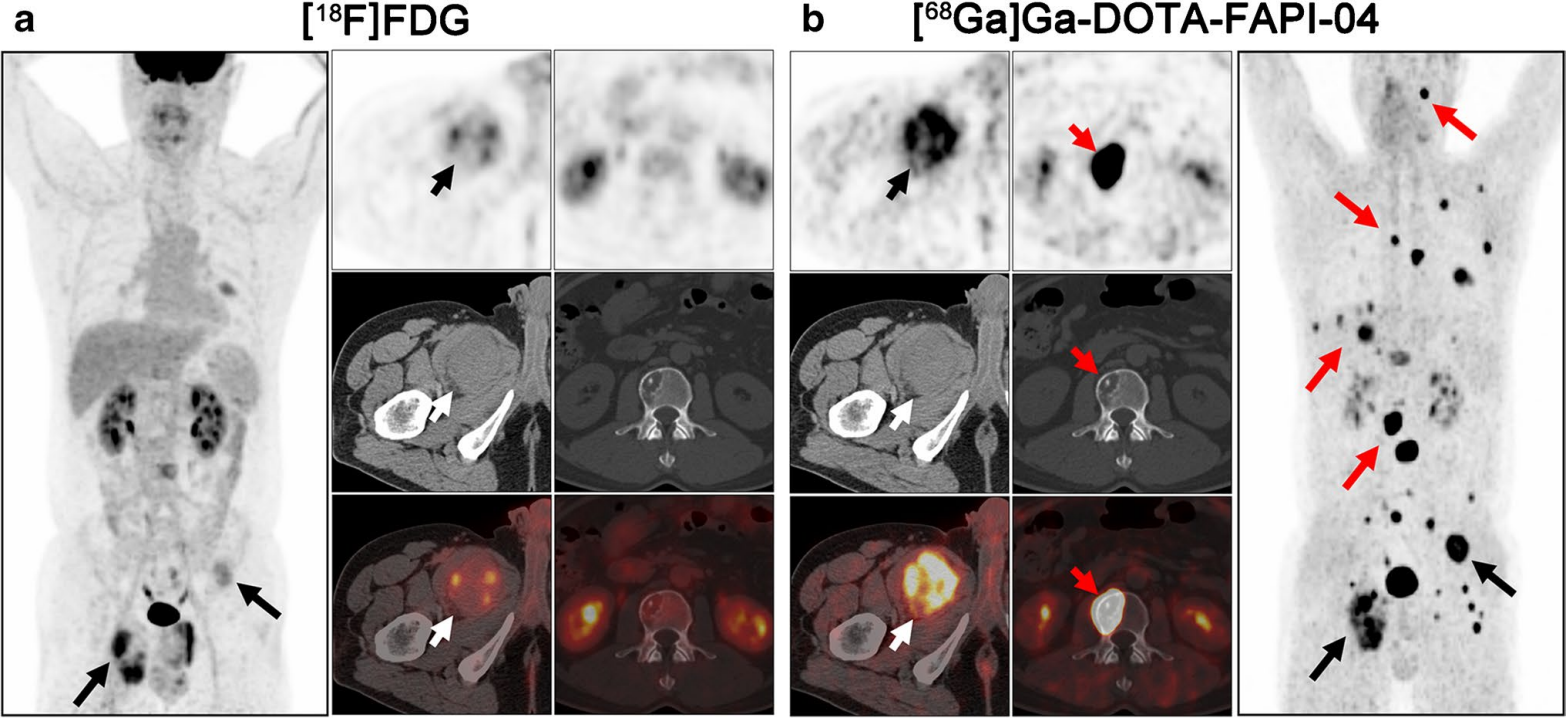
A 47-year-old man (patient #25) pathologically confirmed with malignant solitary fibrous tumor (MSFT) arising from right the thigh received radical operation 1 year ago. [^18^F]FDG PET/CT (a) demonstrated the relapse of right thigh and some bone metastases with low metabolic activity. Compared with [^18^F]FDG, [^68^ Ga]Ga-DOTA-FAPI-04 PET/CT (b) demonstrated more metastases, including lung, bone, and liver metastases. Moreover, all the relapse and metastases showed intensive uptake of [^68^ Ga]Ga-DOTA-FAPI-04. Black and white arrows indicated the tumor lesions detected by both tracers, and red arrows indicated the tumor lesions detected by [^68^ Ga]Ga-DOTA-FAPI-04 alone
